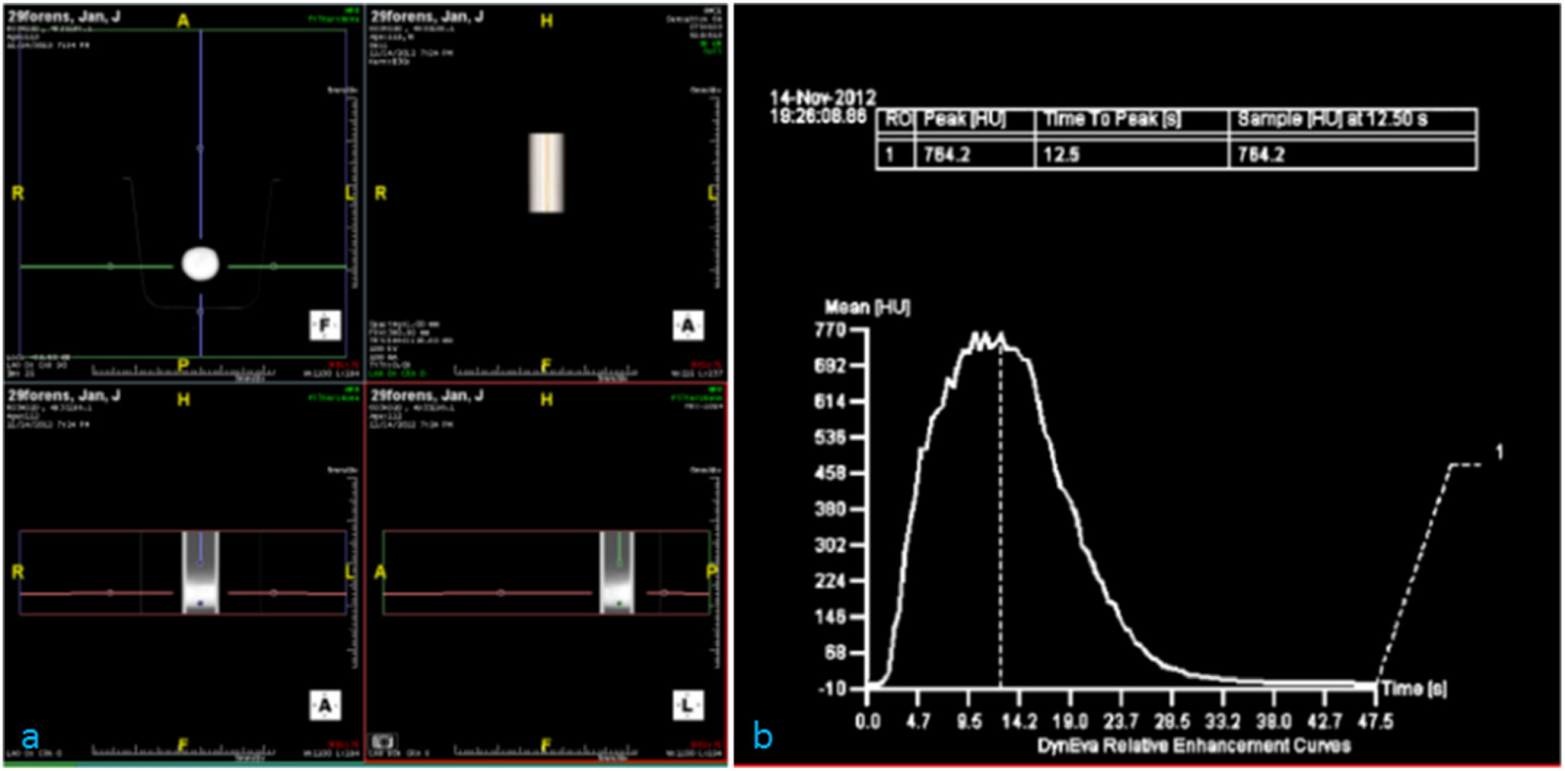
Result of the test with Computed Tomography. The 3D image of the scanned section of tubing (a) and the washout profile (b)
